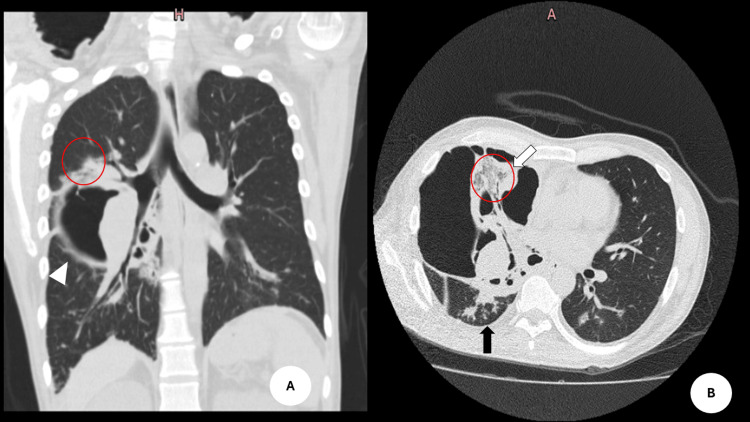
Pulmonary CT scans A: Axial section showing an extensive cavitary lesion in the right middle lobe with thickened walls (white arrowhead). The cavity contains solid soft-tissue components (black arrowhead), with associated findings including an air bronchogram (red circle), bronchial wall thickening, and a tree-in-bud pattern (black arrow), consistent with endobronchial involvement. B: Coronal reconstruction showing the same cavitary lesion, with better visualization of its extent and involvement of the upper pulmonary segments (red circle), as well as bronchial involvement (dotted arrow).
